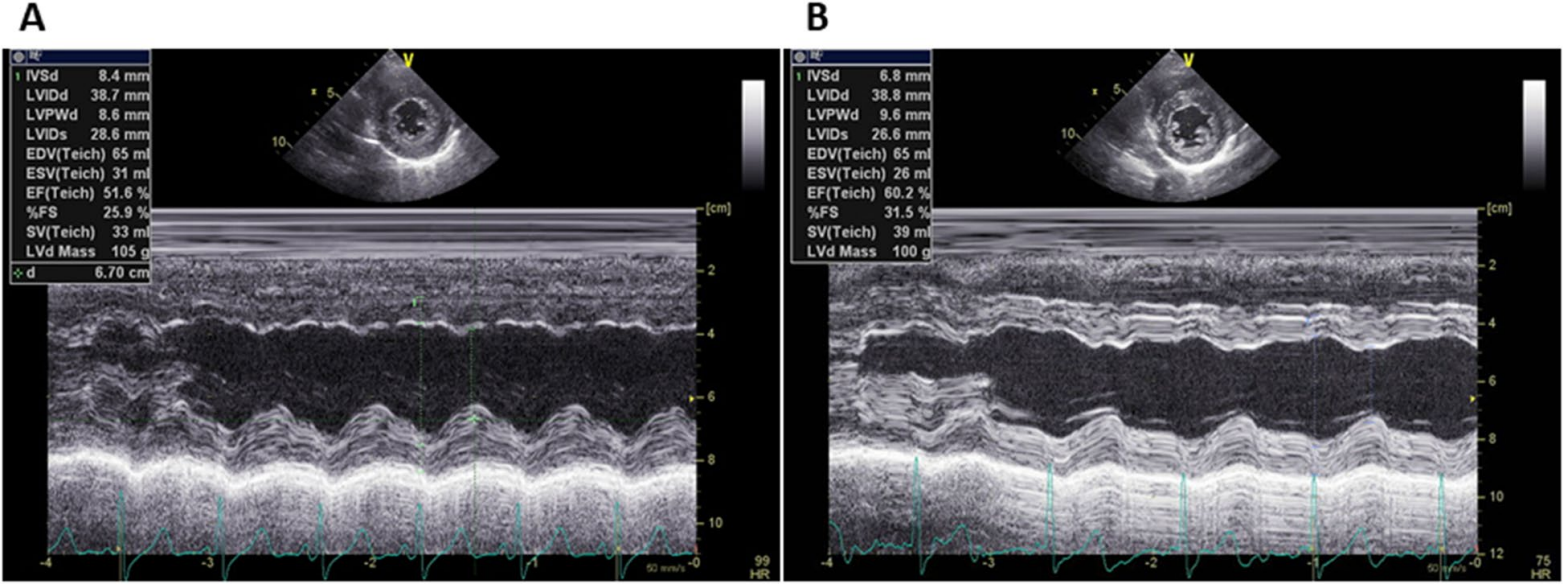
Ultrasound cardiography (UCG) 5 days and 8 days after onset of first headache. (A) Ultrasound cardiography (UCG) 5 days after onset revealed a decrease in wall motion on the left side at the base of the ventricular septum, with a slight decrease in ejection fraction (EF = 51.6%) and in fractional shortening (FS = 25.9%). (B) Eight days after onset, wall motion and EF/FS had improved
